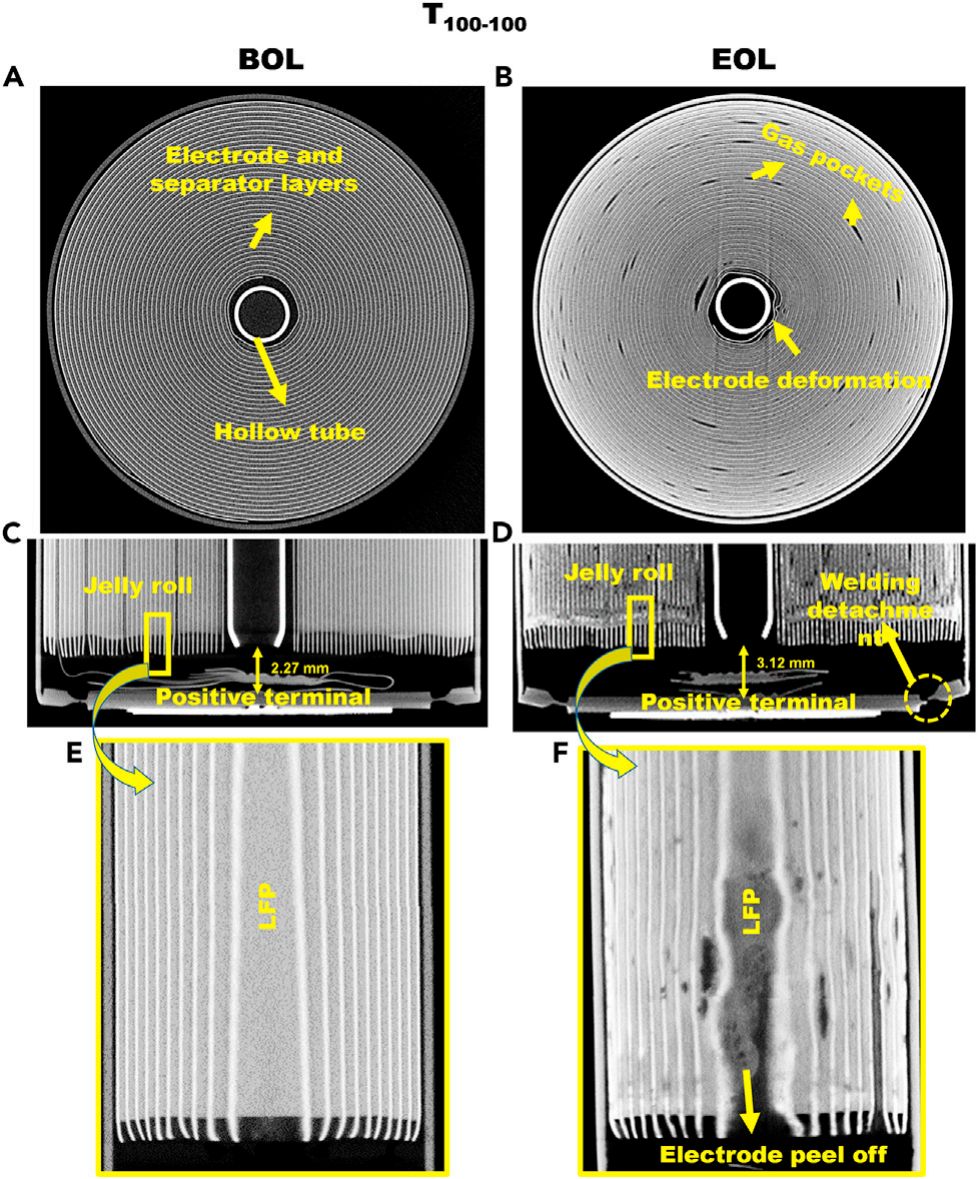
CT scans of T_100-100_ cycled cells at BOL (A, C, E) and EOL (B, D, F)
